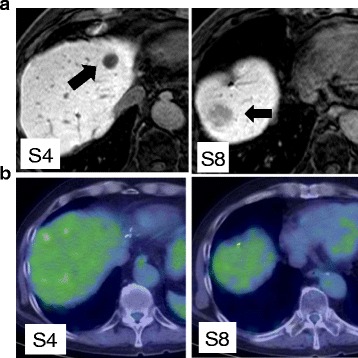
a Gd-EOB-DTPA-enhanced MRI appearance of two liver tumors, in segments 4 and 8. b PET-CT appearance of two liver tumors, in segments 4 and 8
